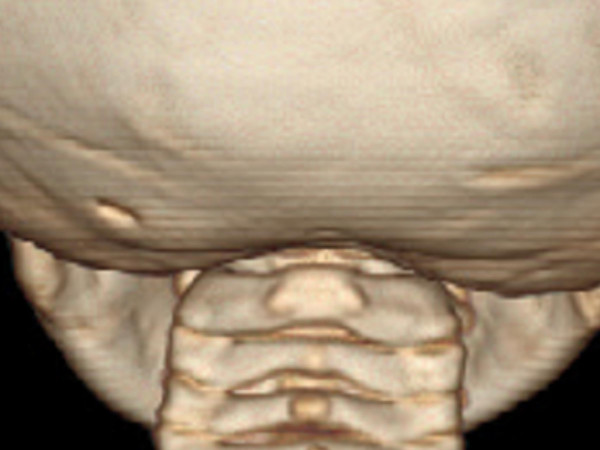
3D reconstruction CT scan showed agenesis of posterior arch of the atlas.
